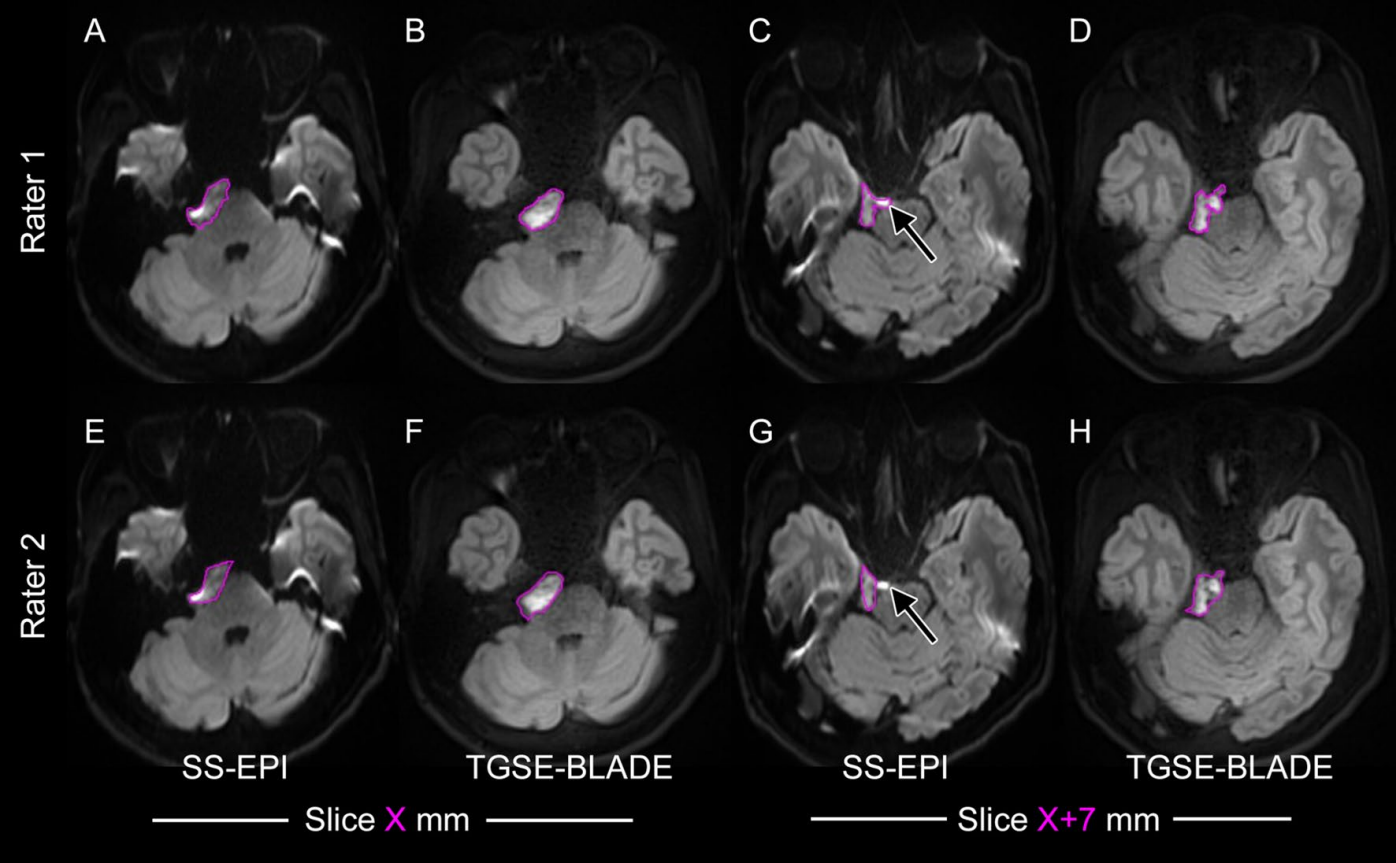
Representative images of the suspected epidermoid cyst at the right cerebellopontine angle. Rater 1 (A, B, C, D) and Rater 2 (E, F, G, H) independently placed ROIs encircling the entire epidermoid lesions on SS-EPI DWIs (A, C, E, G). Subsequently, Rater 1 and Rater 2 placed ROIs in the same manner on TGSE-BLADE DWI (B, D, F, H) 3 weeks later to avoid any learning effect. ROIs for the entire lesion analysis (Slice X mm and Slice X + 7 mm) are shown (magenta). Rater 1 included a hyperintense area in front of the pons on SS-EPI DWI (arrow, C) as the lesion, while Rater 2 did not (arrow, G). A major discrepancy in ROIs between raters was observed on SS-EPI DWI. These ROIs created by Rater 1 and Rater 2 were also used for Dice coefficient analysis
